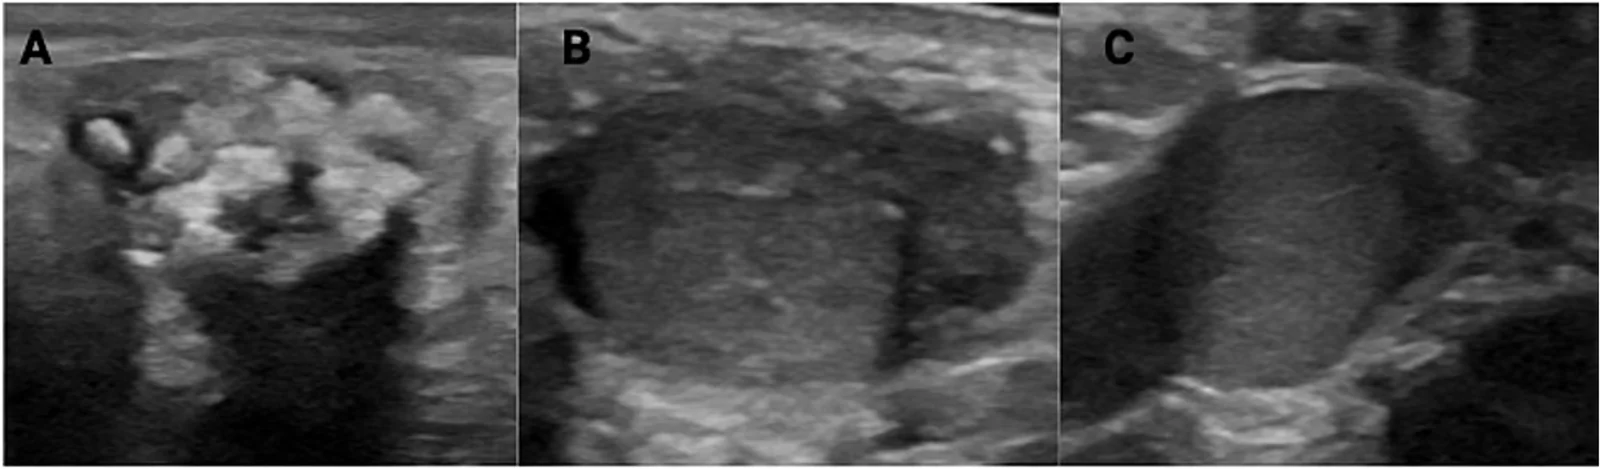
Ultrasound images demonstrating (A) meconium fecalith and (B, C) an otherwise normal appearing right testicle.
